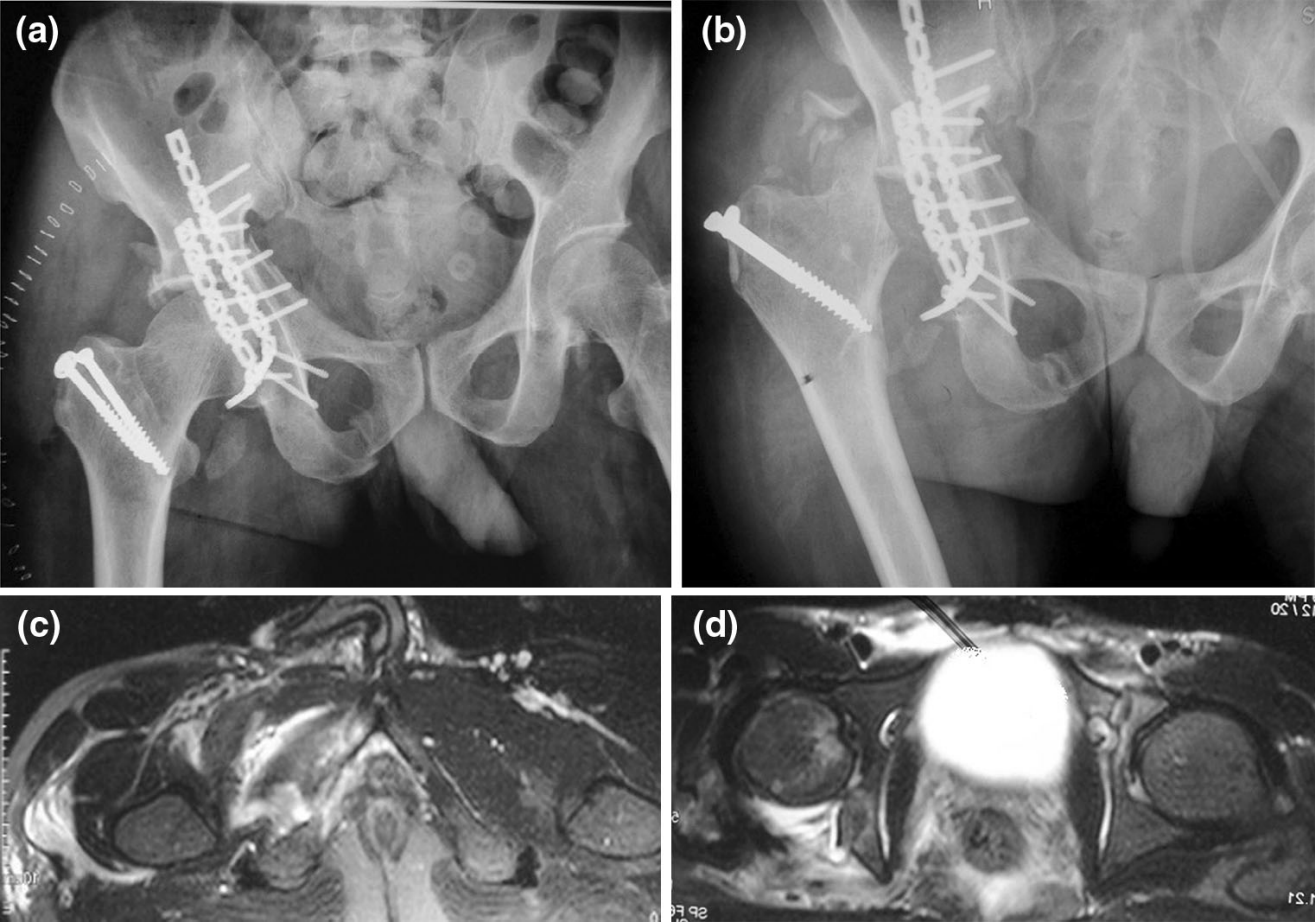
Imaging of case 11 with transverse fracture with posterior wall fracture. a Immediate post-operative antero-posterior radiograph showing
